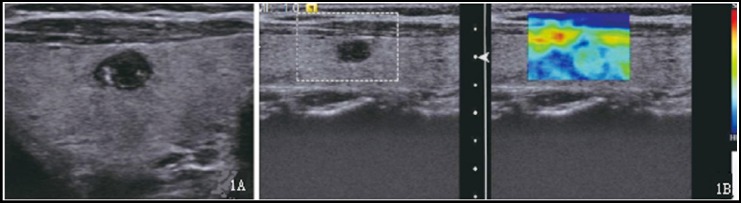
Conventional ultrasound and elastography images of papillary thyroid carcinoma. *Note:* Dorsal nodules in the middle of the thyroid gland, 2A: unclear boundary (1 point), internal iso-echo (1 point), wide or narrow incomplete acoustic halo (2 points), aspect ratio more than 1 (1 point), and internally scattered small strong echoes (2 points), Stacul score: 7 points; 2B: Grade W elastography imaging, pathological result: papillary thyroid carcinoma.
